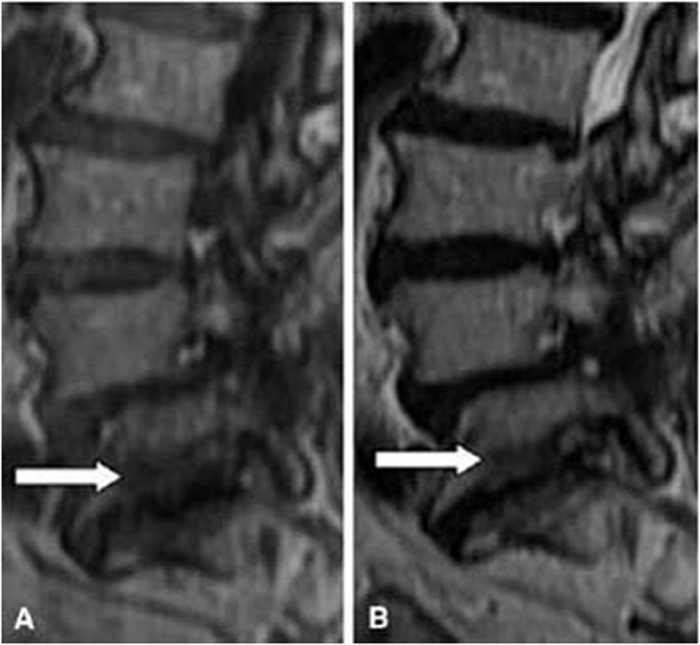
Magnetic resonance images of type III MCs at the L5-S1 level in the lumbar spine.
